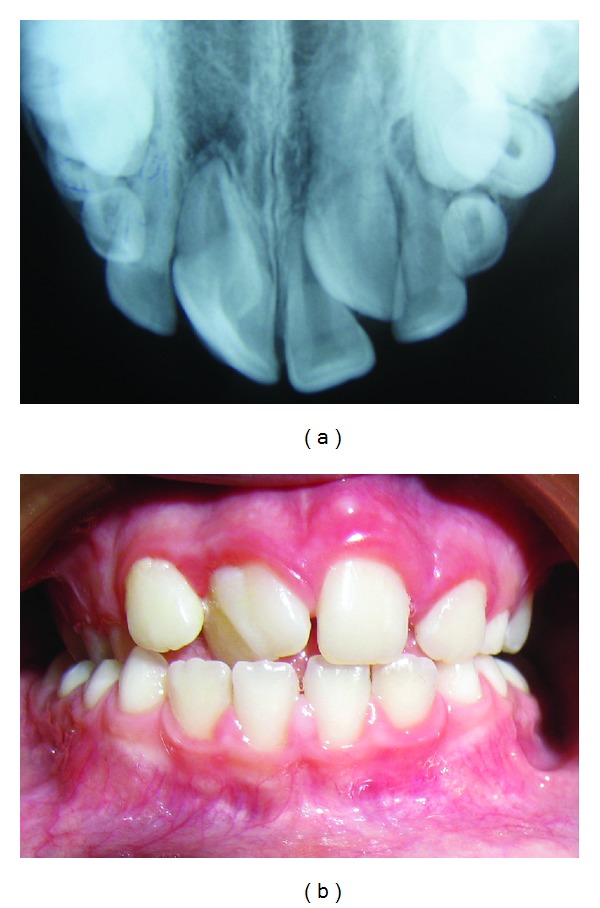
(a) Occlusal radiograph of the patient, demonstrating the large incisor and the unerupted supernumerary incisor. (b) Close-up view of the tooth, showing abnormal crown dimensions and morphology.
